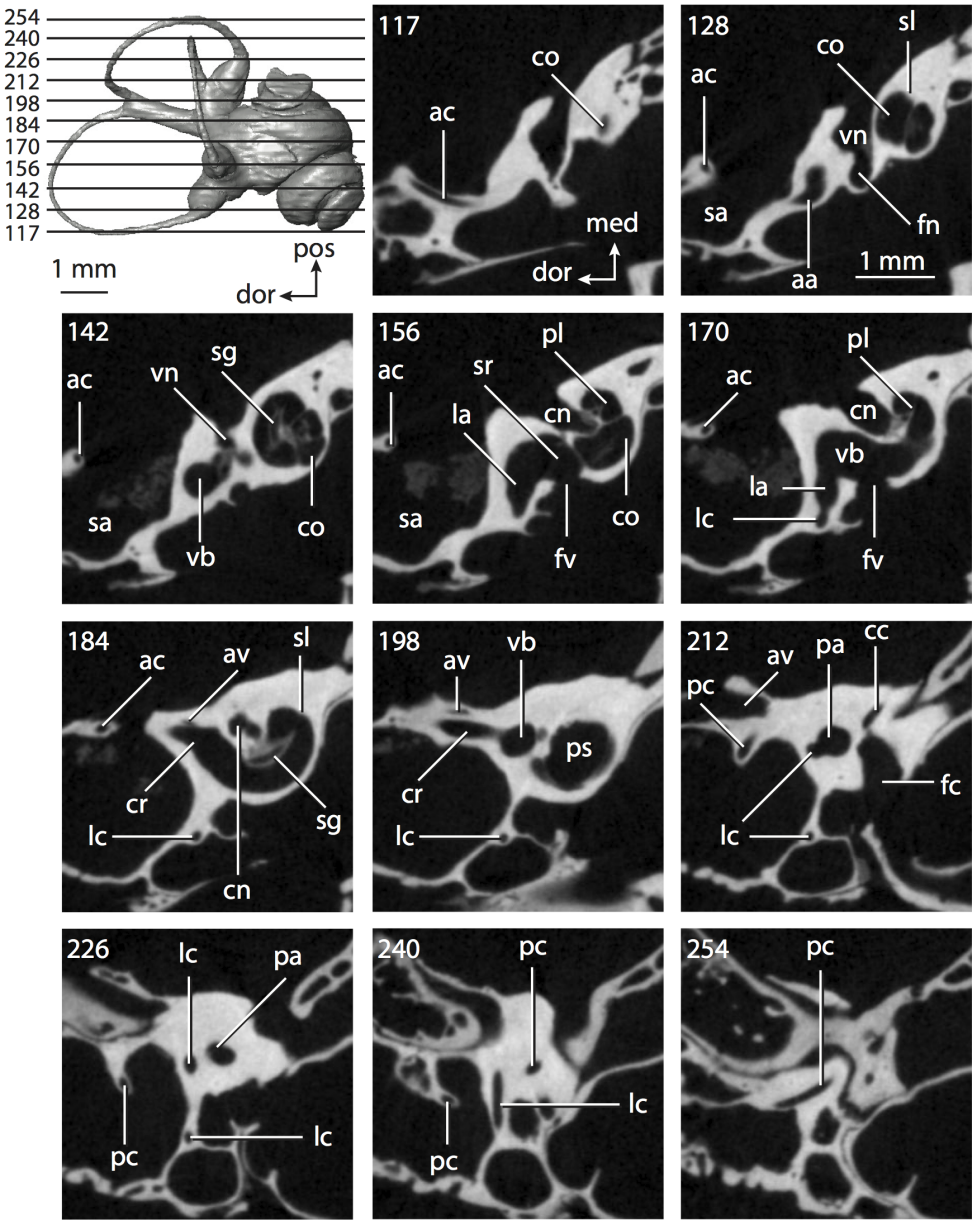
CT slices through ear region of*Sylvilagus floridanus*. Abbreviations listed at the end of the Materials and Methods section.
